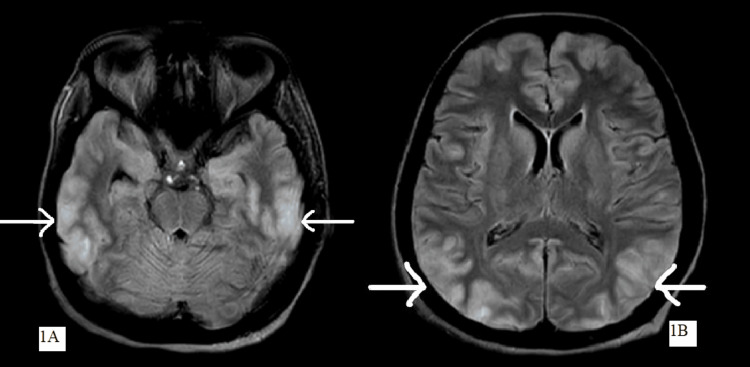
MRI brain showing altered signal intensity with vasogenic oedema in bilateral frontal, temporal and parietal lobes (as shown in Figure 1A) and occipital lobes (as shown in Figure 1B) suggestive of posterior reversible encephalopathy syndrome. MRI: magnetic resonance imaging
